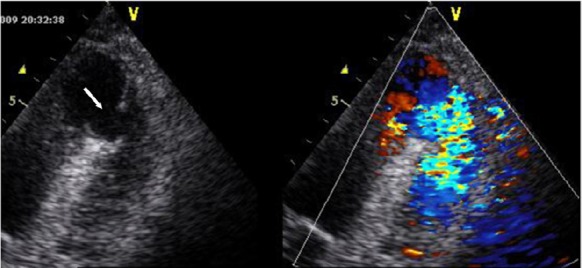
In this patient, because of difficulties in the routine arterial approach, we decided to advance the A_1_ catheter from the femoral vein access to RV, then to LV via VSD. A 0.035 inches ×3 m Amplatz guidewire was advanced to RV crossing VSD into LV, ascending and then descending aorta for better support. The delivery sheath was then advanced from the venous side to the LV cavity over the wire and the dilator and the wire was carefully removed. A 20-mm Amplatzer PIMVSD device (AGA Medical) was used. The occluder device was then delivered to the LV. The device was extruded from the sheath until the LV disc was opened under echocardiographic guidance to ensure that the device did not open in the mitral chordal apparatus (Figure 2A). It was then withdrawn toward the interventricular septum. After further satisfactory echocardiographic evaluation of septal alignment, the RV disc was also deployed and the device was released from the delivery cable (Figure 2B).
